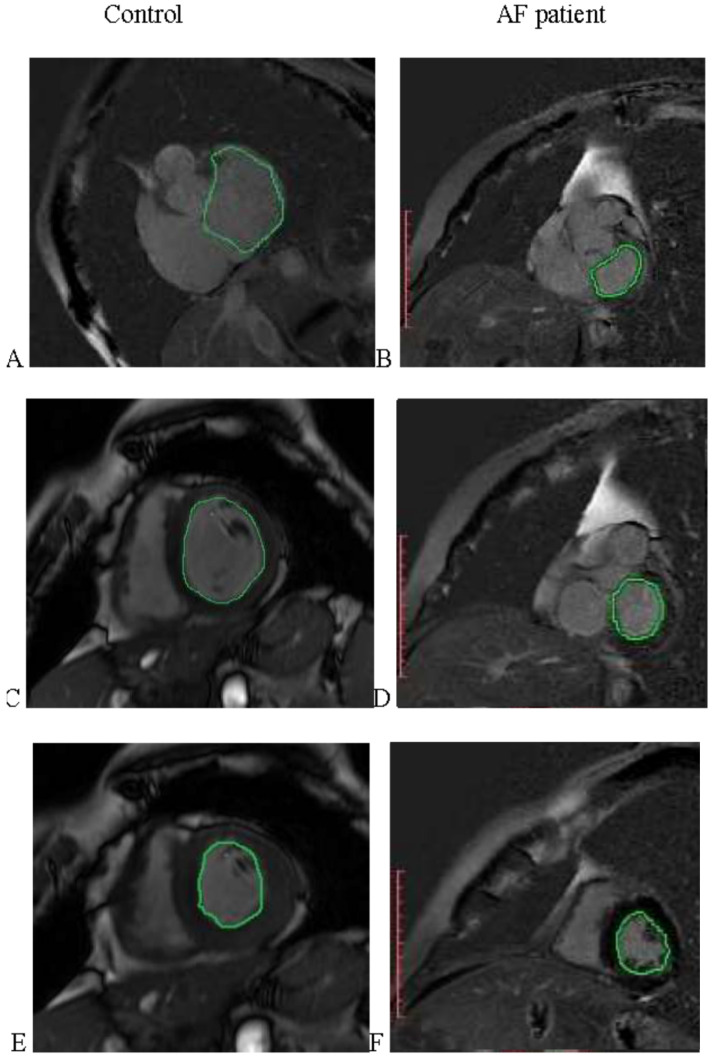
Representative cardiac MRI images from a healthy subject (Control—left panel) and from an AF patient (right panel) used to determine LA Vol (A,B), EDV (C,D), and ESV (E,F), respectively.
